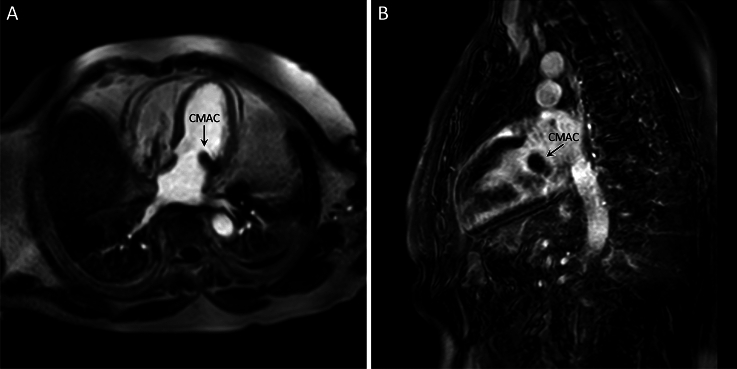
Cardiac magnetic resonance revealing a T1- and T2-weighted hypointense, calcified caseous formation of the mitral valve annulus, without early or late contrast enhancement. *CMAC* caseous mitral annular calcification
